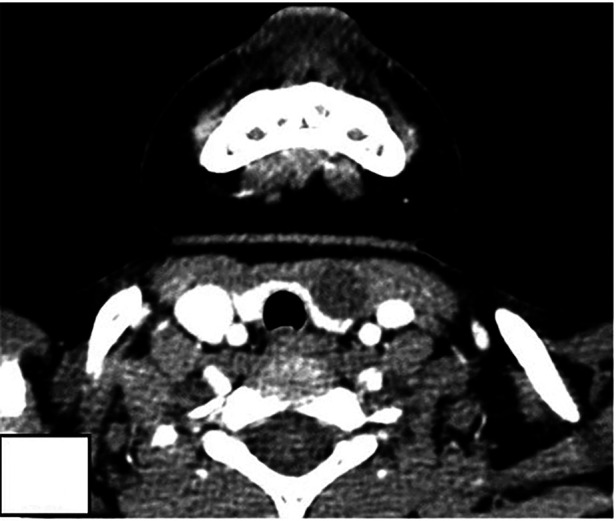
A 3.3-year-old Male. CT showed a 1.5-cm sized homogeneous, low-density mass in the left neck region (white arrow), and located anterolaterally of thyroid. CT showed that the left thyroid was compressed and deformed. This case was misdiagnosed as lymphangioma preoperative.
